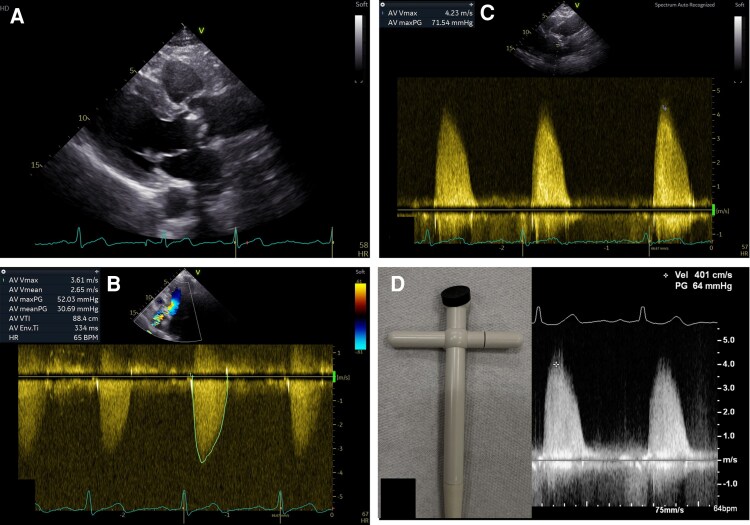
Impact of insonation angle and pressure gradients in aortic stenosis. (*A*) shows a highly calcified bicuspid aortic valve with a Δp max. of 30.7 mmHg obtained in the apical chamber view (*B*). A modified left parasternal long axis view shows a Δp max. of 71.5 mmHg (*C*), whereas pencil probe interrogation at the right parasternal view yields a Δp max. of 64 mmHg (*D*).
